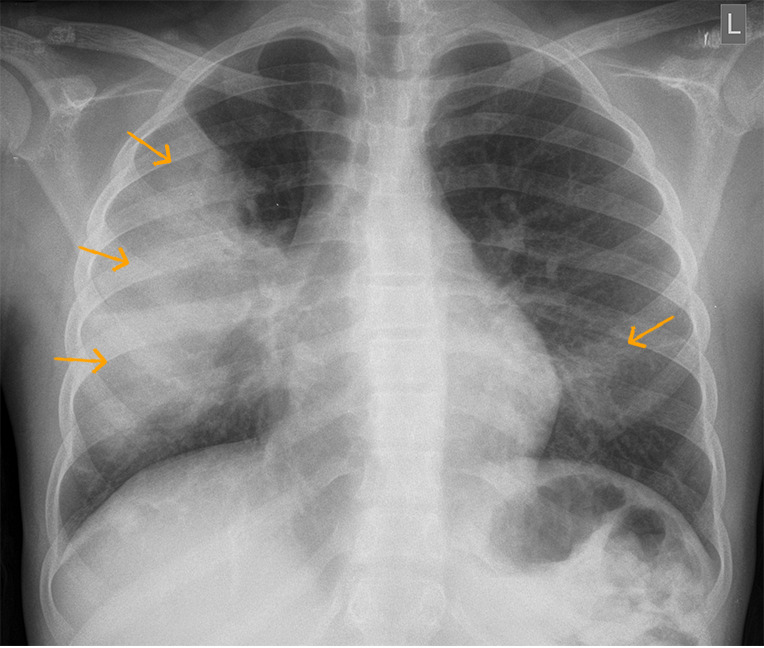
chest X-ray-lung fields showing a large right mid zone consolidation (arrows right) with no significant loss of volume; few left lower zone infiltrates are noted as well (arrow left)
